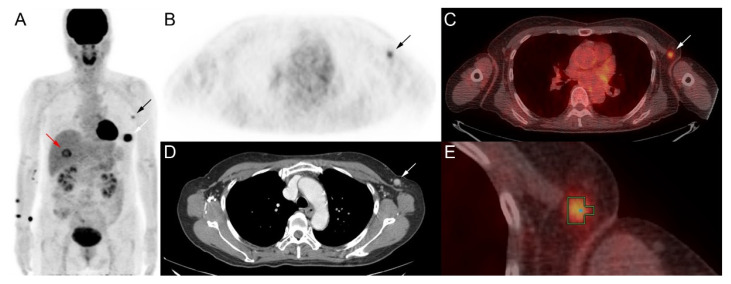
A 60-year-old woman with invasive ductal carcinoma of the left breast. The patient had biopsy-proven metastases of the left axillary lymph node and liver. Maximum intensity projection image of ^18^F-fluorodeoxyglucose positron emission tomography (^18^F-FDG PET) (A) shows abnormally increased ^18^F-FDG uptake in the left breast (white arrow), left axilla (black arrow) and liver (red arrow). Trans-axial PET image (B), fused positron emission tomography/computed tomography (PET/CT) image (C), and contrast-enhanced CT image (D) show an enlarged lymph node with increased ^18^F-FDG uptake in the left axilla (arrows). Volume of interest was drawn around the left axillary lymph node with a threshold of 50% maximum standardized uptake value on fused PET/CT image (E). The maximum standardized uptake value and total lesion glycolysis of the left axillary node were 2.51 and 1.16, respectively. The patient survived without progression during the follow-up period of 33 months.
